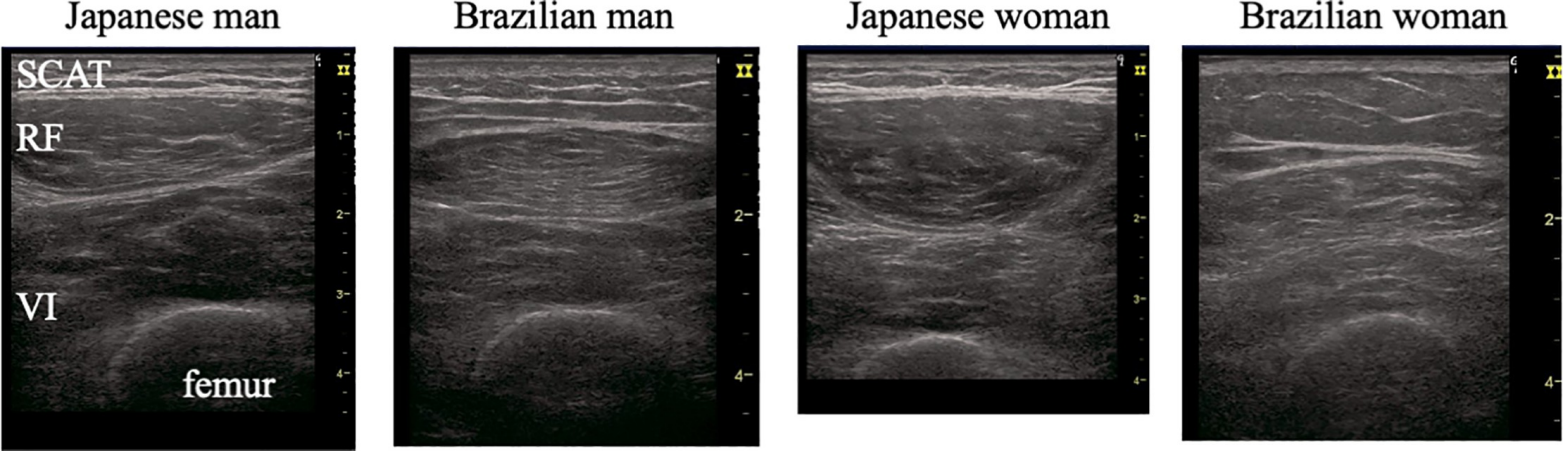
Representative B-mode ultrasound images at the anterior mid-thigh of Japanese and Brazilian older men and women. RF, rectus femoris; SCAT, subcutaneous adipose tissue; VI, vastus intermedius. The ultrasound images were taken with the internal RF tendon located at the center of each image.
